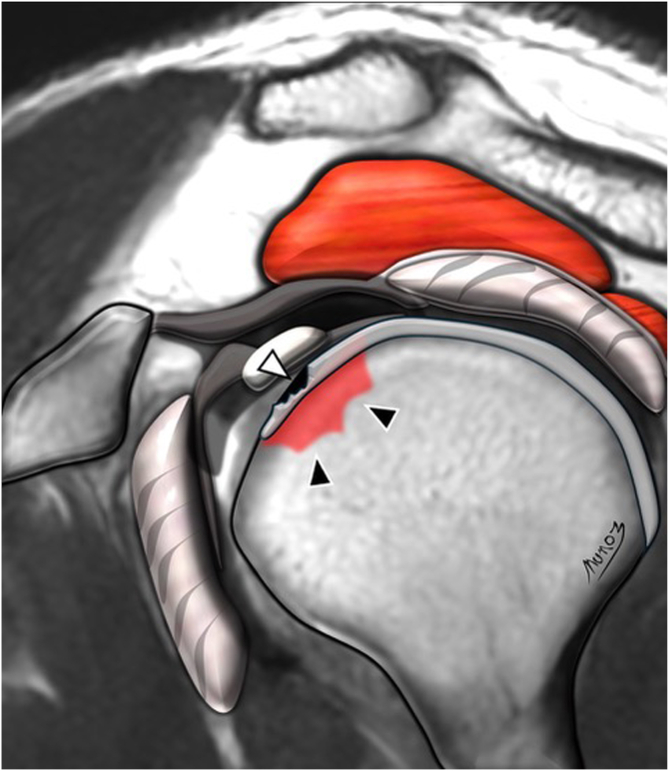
Chondral print sign (white arrowhead) and humeral head subchondral bone edema (black arrowheads) are shown in a sagittal view of a T1-weighted right shoulder MRI. (MRI, magnetic resonance imaging.)
